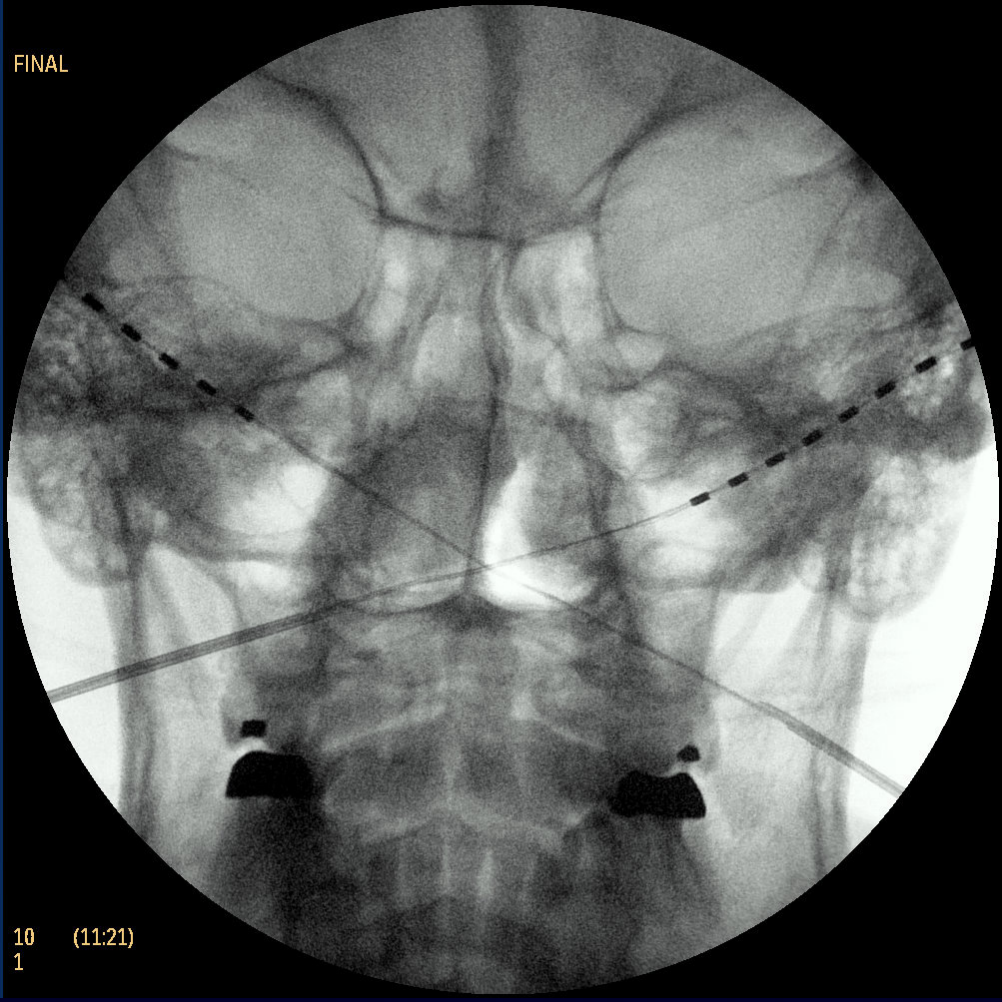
Placement of two octapolar stimulating array leads over the bilateral occipital areas. Placement was carried out with ultrasound guidance and fluoroscopy. The C1-C2 interspace, which serves as a key fluoroscopic landmark for occipital peripheral nerve stimulation, and the dens are easily identifiable.
